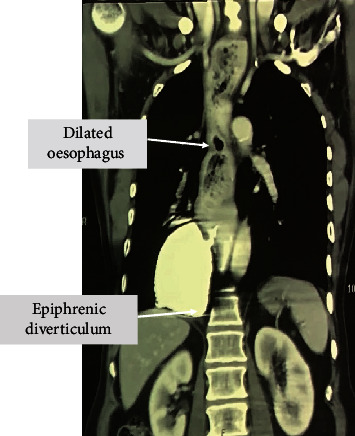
Preoperative CT scan showing oesophageal diverticulum and dilated oesophagus.
